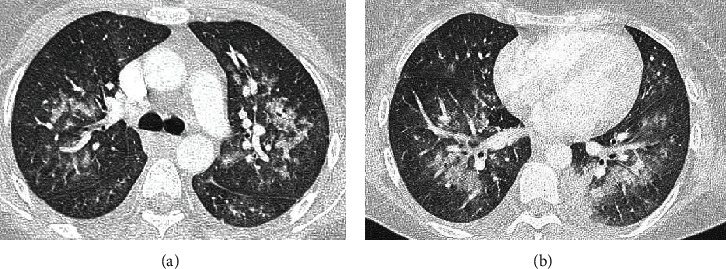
(a, b) CT images of hemorrhagic alveolar pneumonia at its onset at ICU admission.
